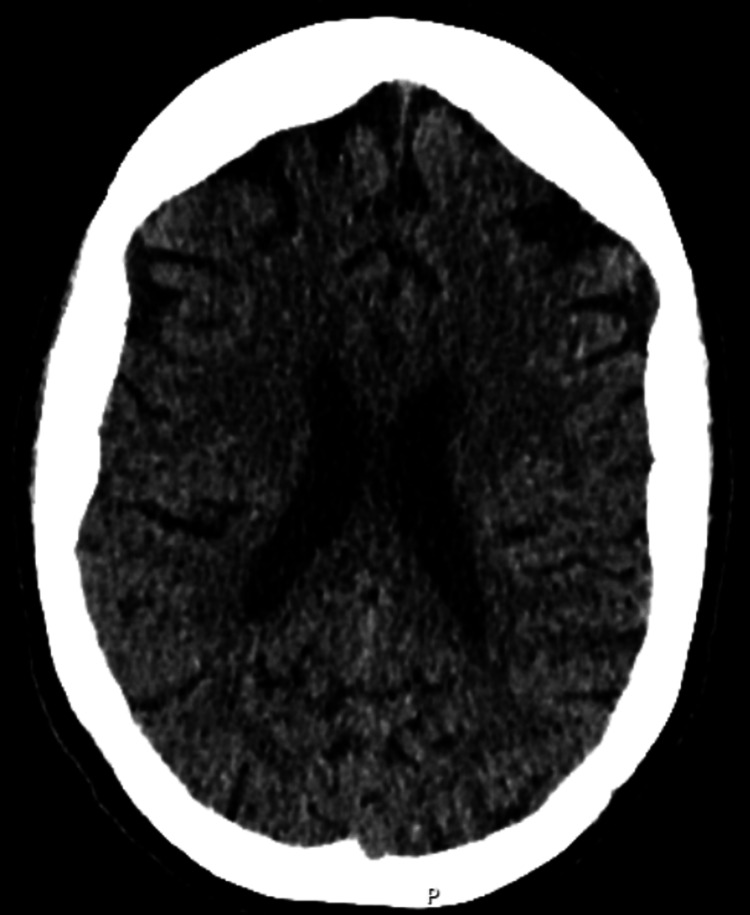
CT brain with no evidence of intracranial lesion or pathology CT: computed tomography
